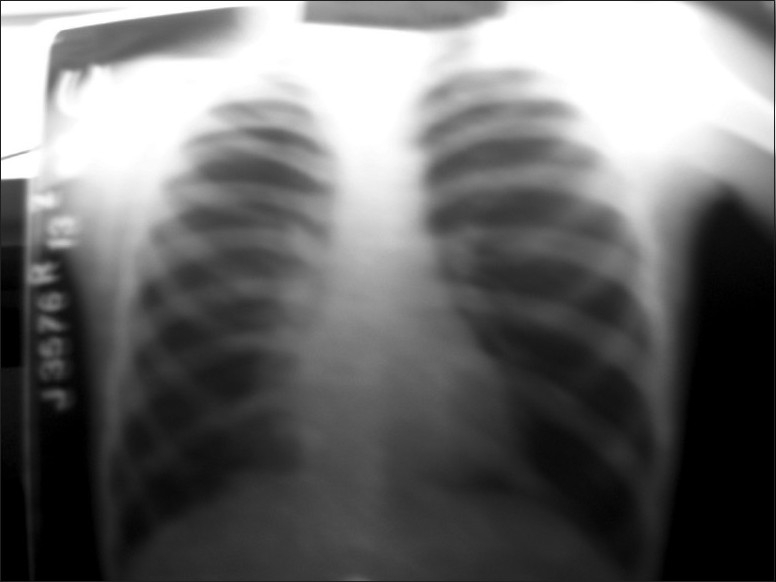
Chest x-ray showing emphysema.
